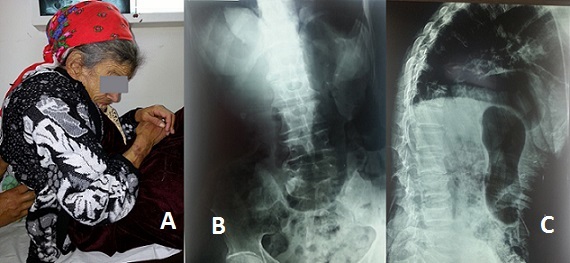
(A) anté flexion de la tête et du tronc; (B) radiographie du rachis dorsolombaire de face: inclinaison droite du rachis sans rotation des corps vertébraux; (C) radiographie du rachis dorsolombaire de profil: effacement de la lordose lombaire, exagération de la cyphose dorsale
